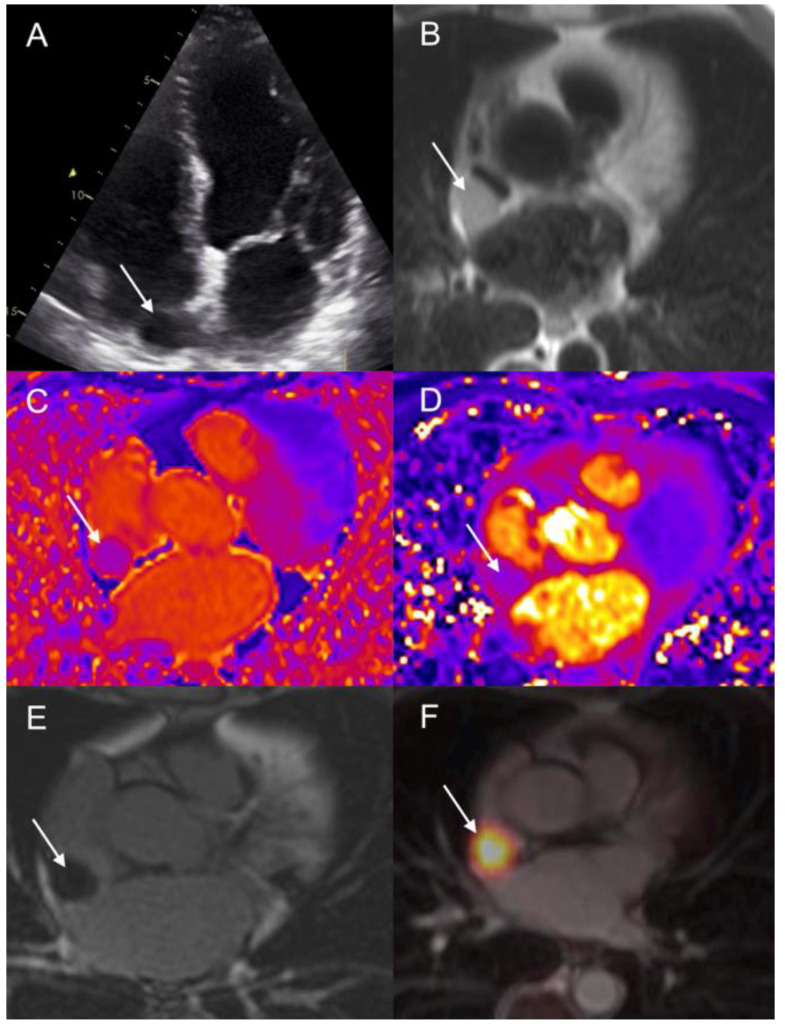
Patient 4. TTE (4-chamber view) showing hypoechogeneous lesion on the right side of inter-atrial septum ((A) arrow). Lesion showed intermediate signal intensity on T1-weighted dark-blood CMR images ((B) arrow). T1- (C) and T2-mapping (D) analysis revealed short T1 and moderately increased T2 values (D). CM showed a slight peripheral enhancement on delayed post-contrast inversion recovery sequences (E). Fused ^68^Ga-DOTATOC PET/CMR images (axial slice) (F).
